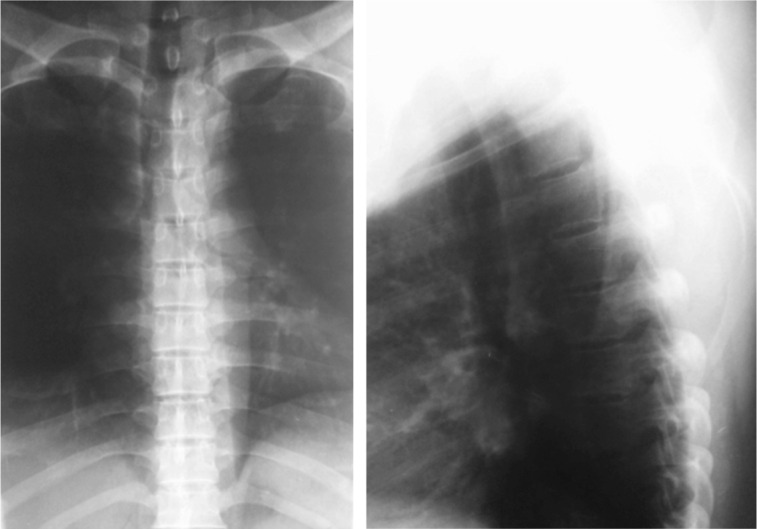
Radiographs of the thoracic spine are normal.
